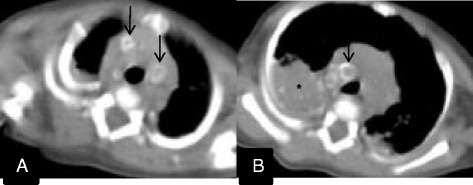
Follow-up chest CT at 5 months of age. A: There was uniform rim calcification of multiple enlarged lymph nodes in the mediastinum (black arrows). B: Dense mass-like consolidation of the bilateral lower lobe of the lungs remained, with multiple fine spots of calcification (star).
